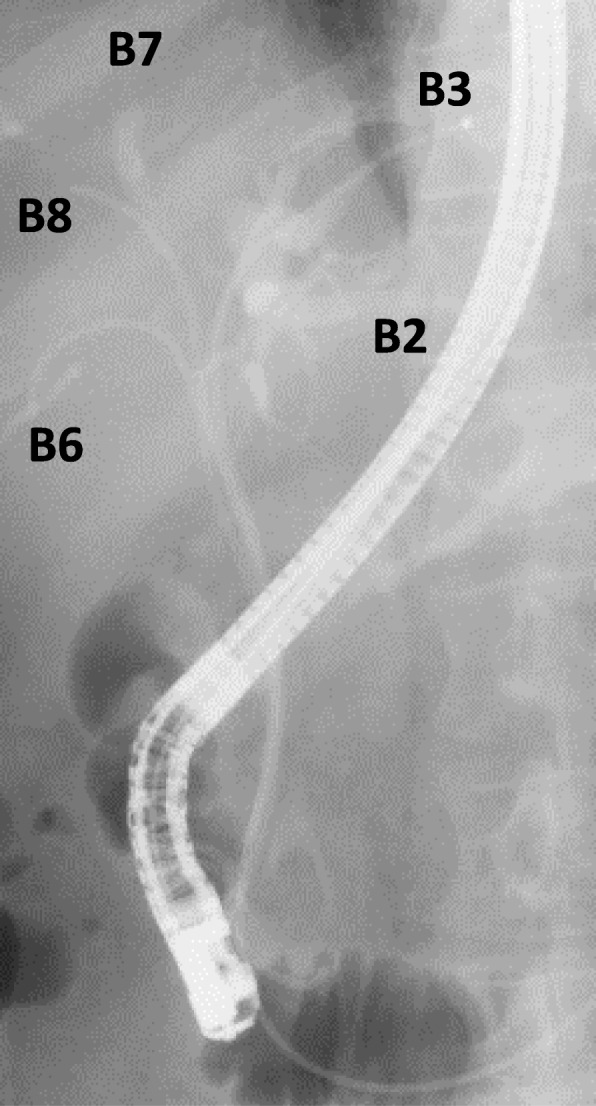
A 2D X-ray image of replaced PSs. PS, plastic stent; 2D, two-dimensional
